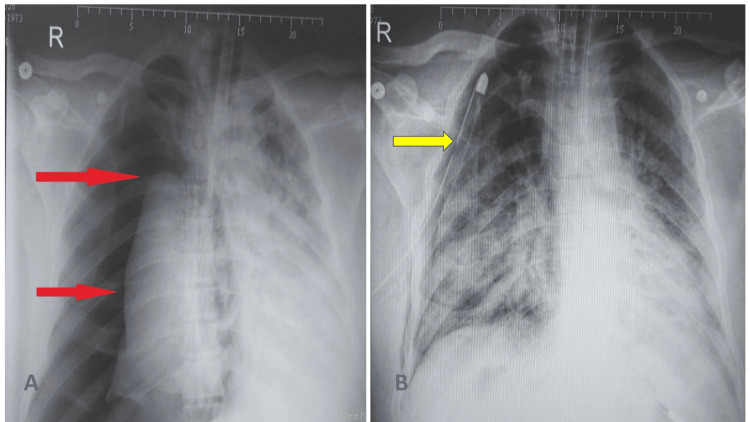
(A) Tension pneumothorax in a mechanically ventilated patient and (B) chest radiograph showing the placement of the chest tube The red arrows in Panel A indicate the pneumothorax, and the yellow arrow in Panel B indicates the chest tube.
